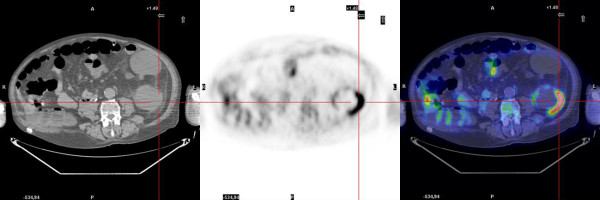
FDG-PET scan: intracystic kidney infection in a CKD patient with multiple cysts.
